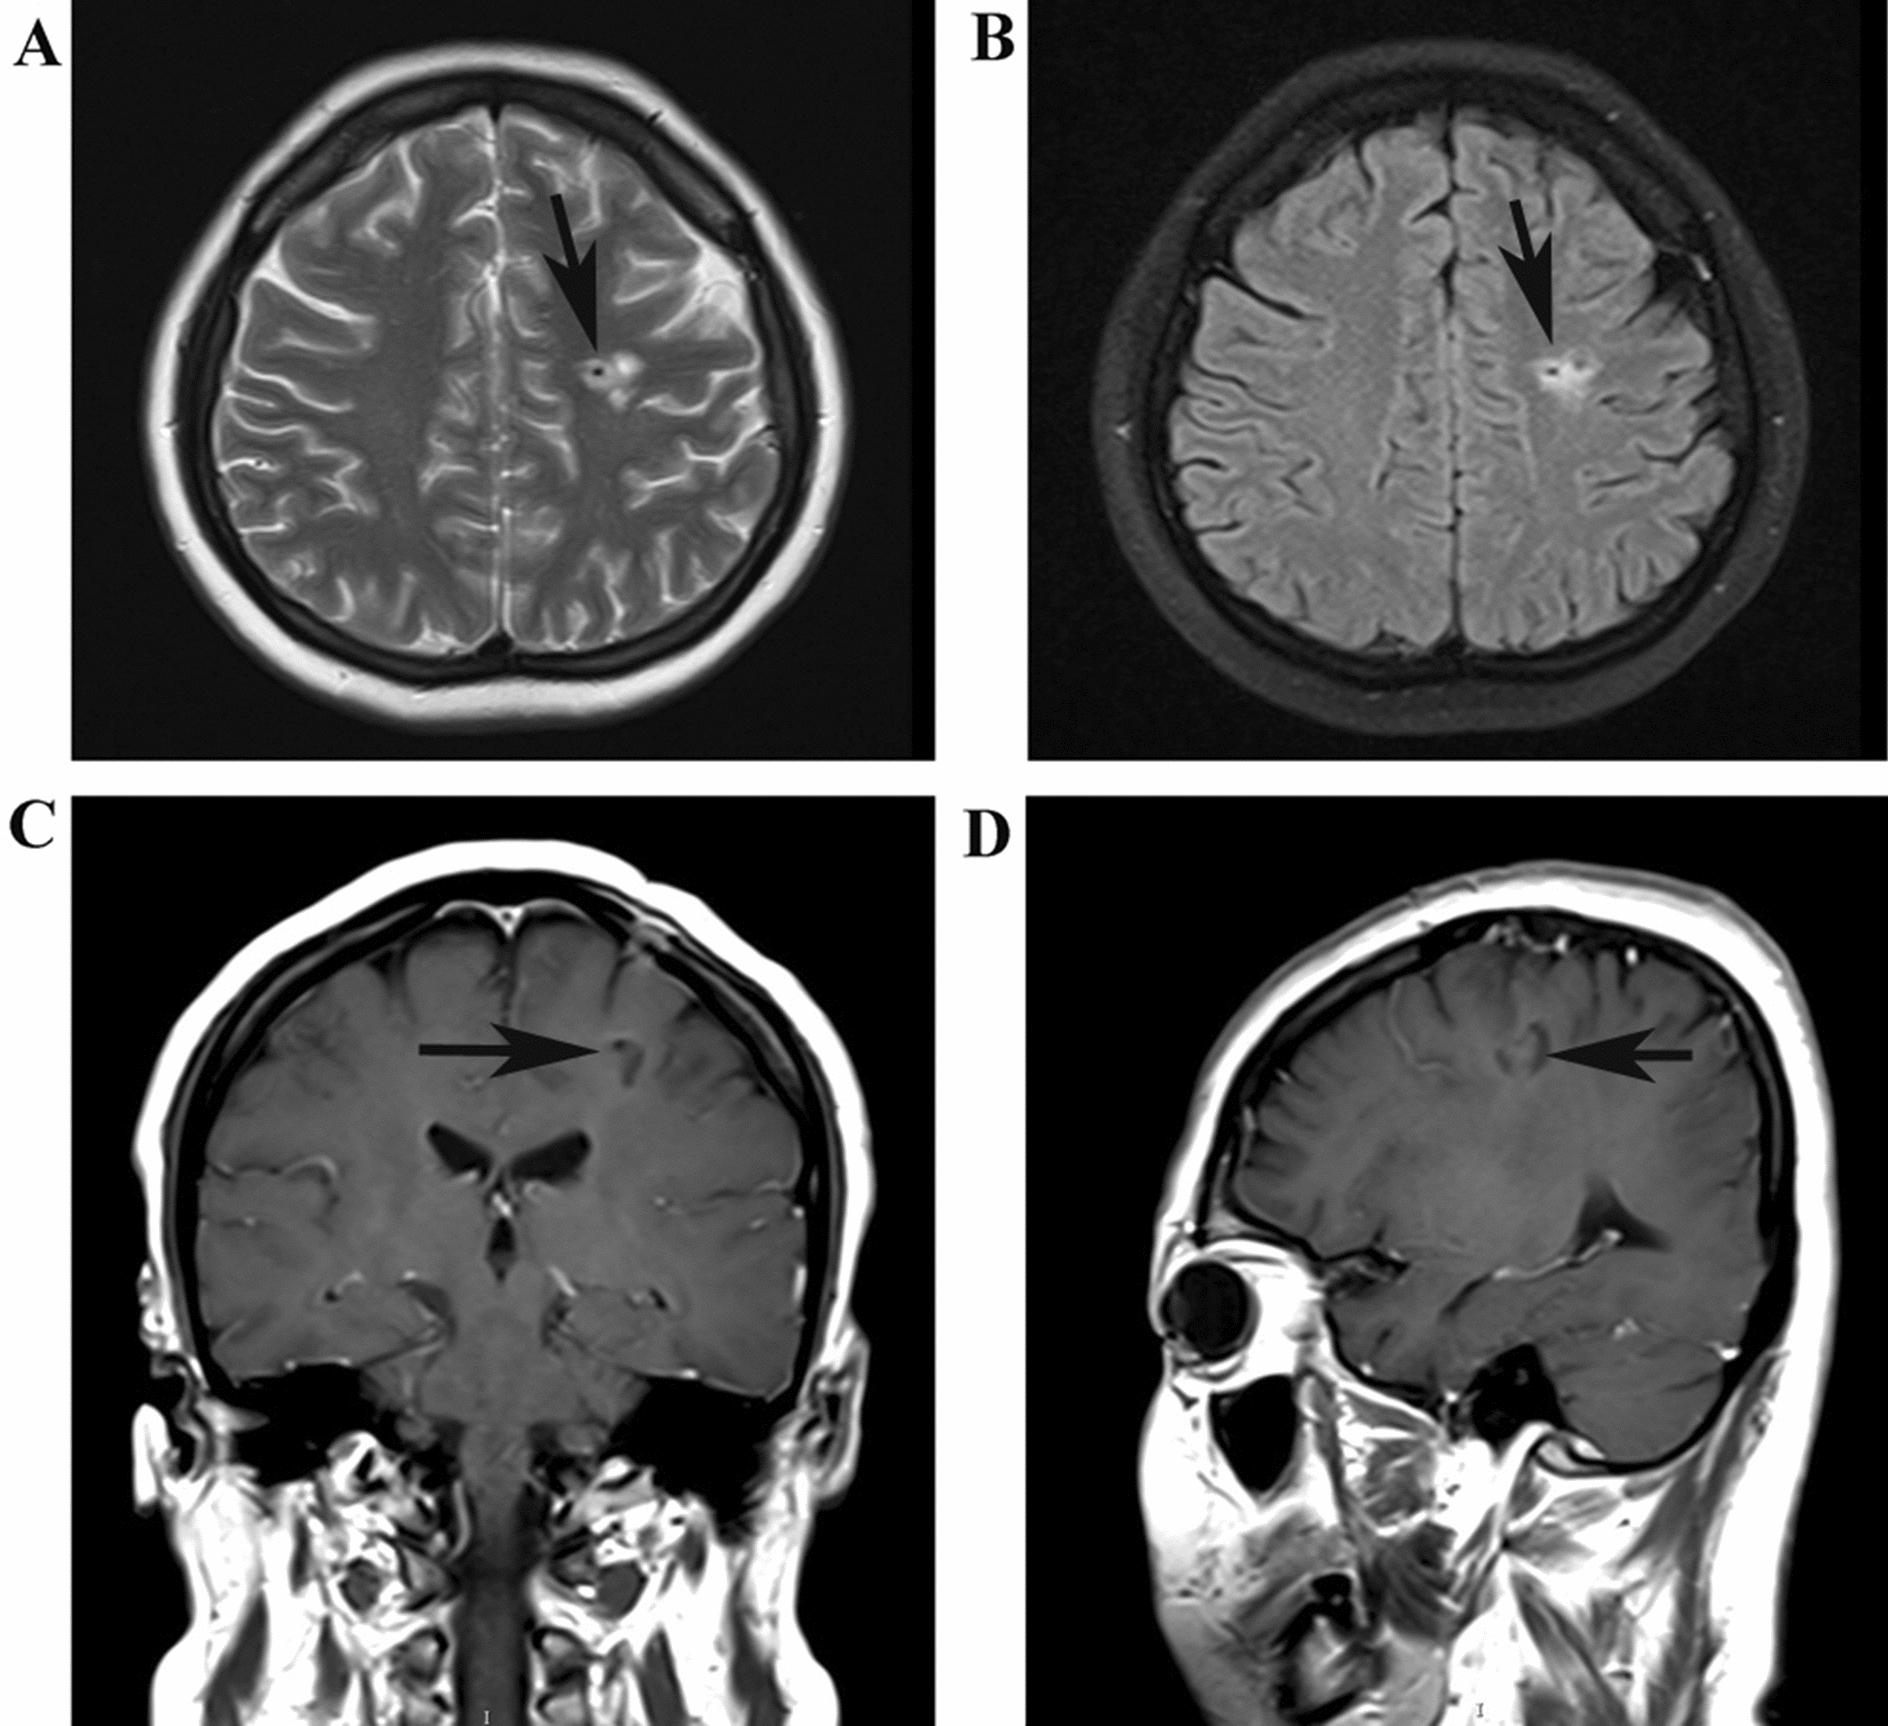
Magnetic resonance images obtained 2 months after surgery showed a small residual cavity of hypointensity on T1-weighted imaging (T1WI) and hyperintensity on T2-weighted imaging (T2WI) and fluid-attenuated inversion recovery (FLAIR), with a clear boundary in the left centrum semiovale after surgical treatment with no enhancement (arrows point to the center of the focus). A T2WI axial view. B T2-FLAIR axial view. C Contrast enhanced (CE)-T1WI coronal view. D CE-T1WI sagittal view
